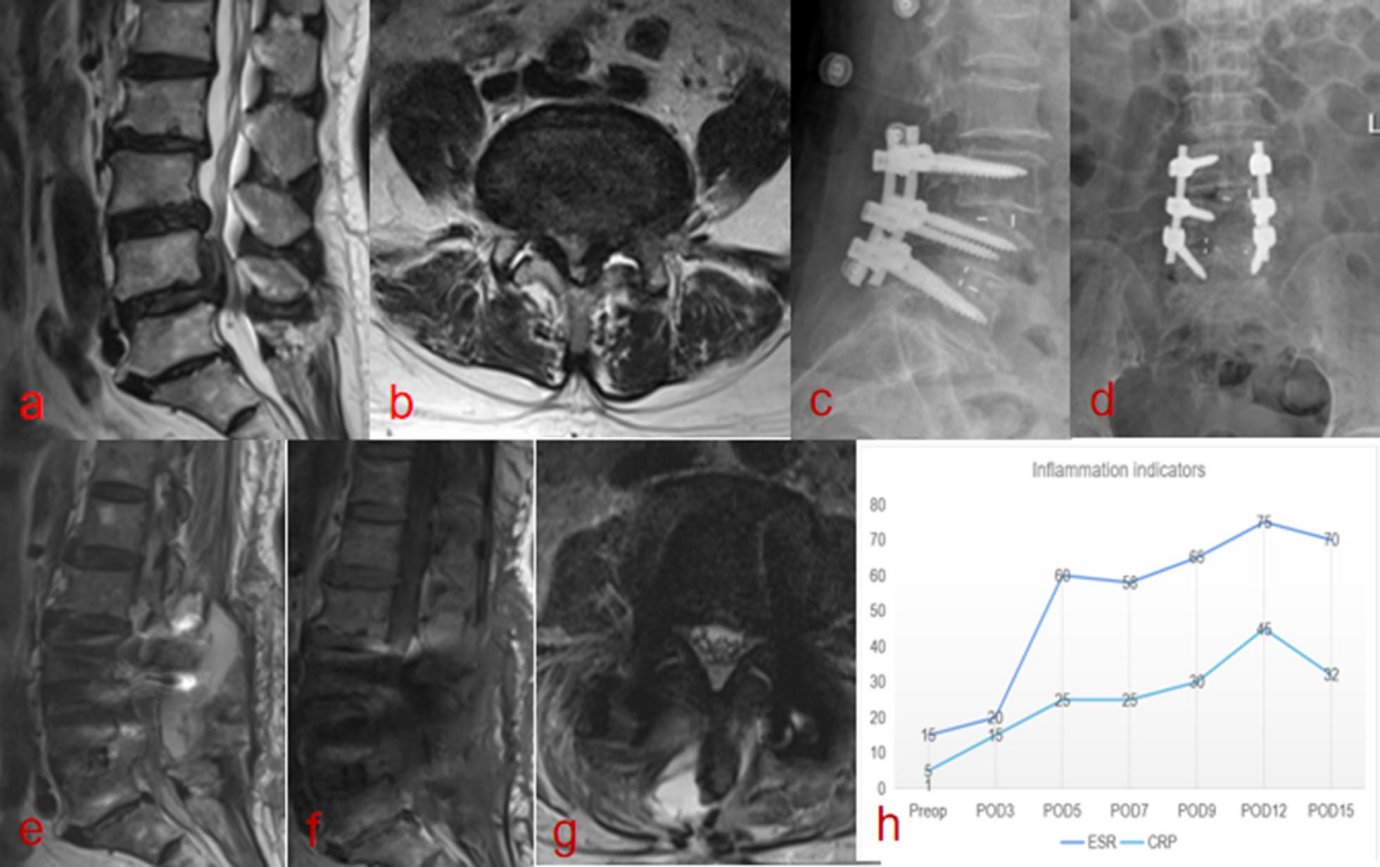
A 57-year-old male patient with postoperative surgical site infection. a, b Lumbar MRI showed preoperative disk herniation and spinal canal stenosis. c, d Lumbar postoperative X ray showed satisfactory position of screw and cage. e, f On the 4th day after surgery, T2WI sagittal plane and cross-sectional plane showed mixed high and equal signals and g T1WI showed a low signal in the deep incision. h serum C-reactive protein (CRP) level and erythrocyte sedimentation rate increase sharply from postoperative day 3. The SSI was confirmed by his complaint of localized pain, symptoms of fever, imaging test and laboratory
